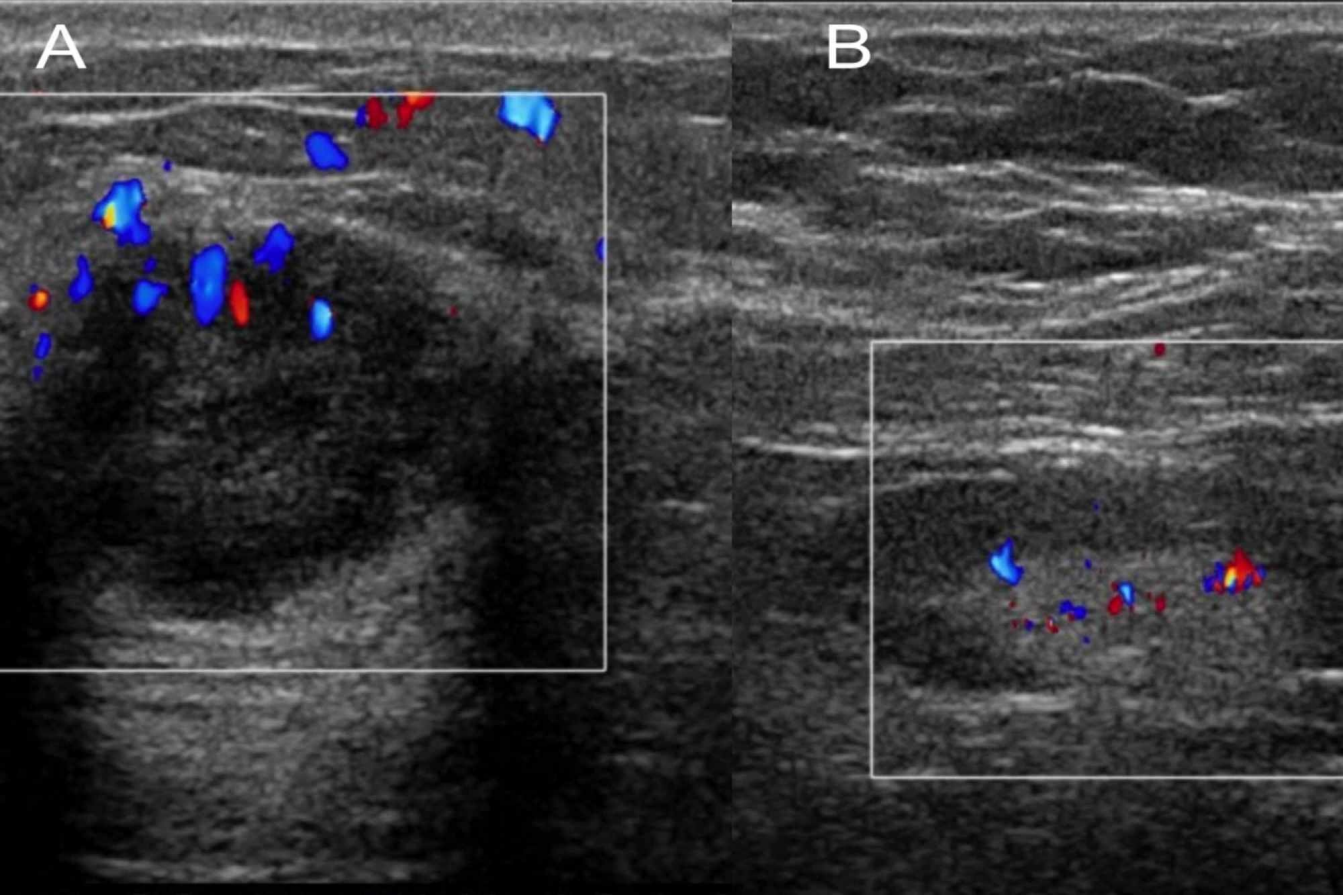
Ultrasound of the right breast and axilla. The ultrasound of the right breast (A) and axilla (B) demonstrates a 1.6 x 1.3 x 1.8 cm microlobulated hypoechoic mass. Several abnormal lymph nodes were noted within the right axillary region with abnormally thickened cortices.
